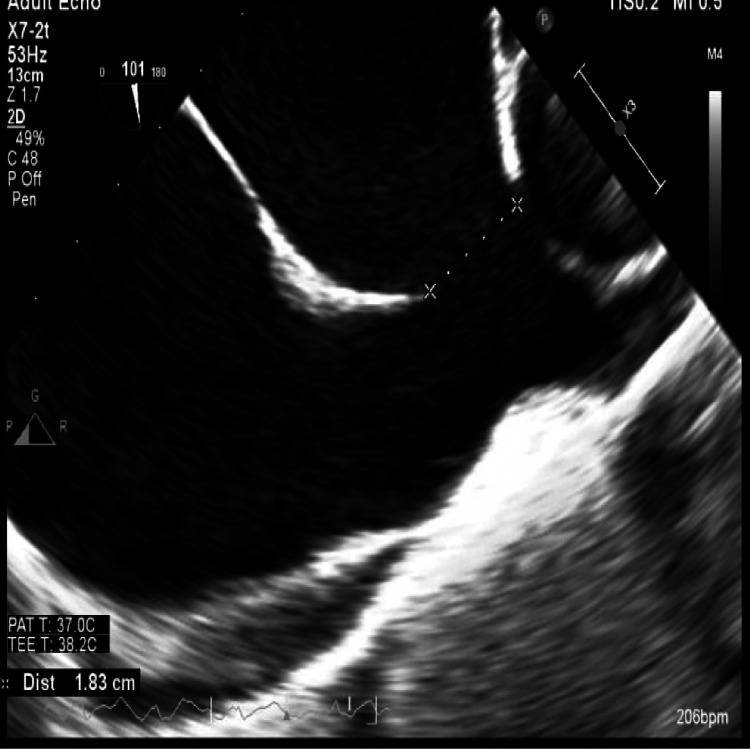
A grayscale image from a TEE shows a 1.83 cm superior SVASD. TEE: transesophageal echocardiogram, SVASD: sinus venosus atrial septal defect.
